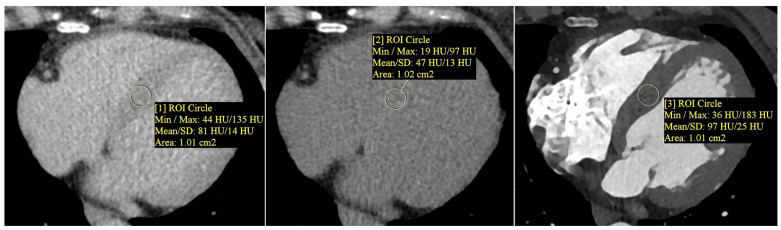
Measurement of myocardial and blood pool attenuation (Hounsfield units, HU) was performed by placing circular regions of interest (ROIs, about 1.0 cm^2^) on native and delayed-phase cardiac CT images to quantify extracellular volume (ECV). From left to right: native (non-contrast) myocardium—ROI [1]; delayed-phase myocardium—ROI [2]; delayed-phase blood pool—ROI [3]. ROIs were positioned to avoid trabeculae, epicardial fat, and calcifications. Mean HU values ± SD are displayed. ECV was calculated using a standard formula that incorporates the patient’s hematocrit and the attenuation difference between the myocardium and blood pool. Note: ROI numbers [1], [2], and [3] refer to the image annotations and do not indicate literature references.
